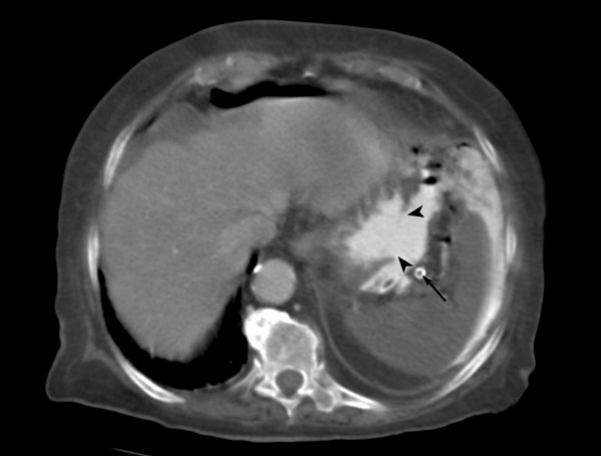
Contrast-enhanced spiral CT shows a large gap at the stomach wall (arrowheads) with lack of enhancement and spillage of oral contrast in the peritoneal cavity. The spleen is uniformly hypodense with thrombus (arrow) in the lumen of the calcified splenic artery. A moderate amount of perihepatic fluid and free peritoneal air are also present.
